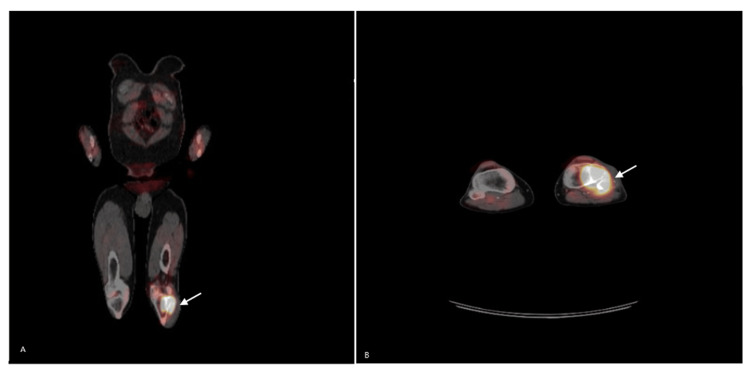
PET scan showing uptake in the left knee A: Osteolytic mass in the left lower knee involving the tibia and fibula with an SUV of 26, indicating high metabolic activity B: A magnified view of the mass in the left lower knee with increased uptake involving the tibia and fibula, along with adjacent soft tissues SUV: standardized uptake value; PET: positron emission tomography
